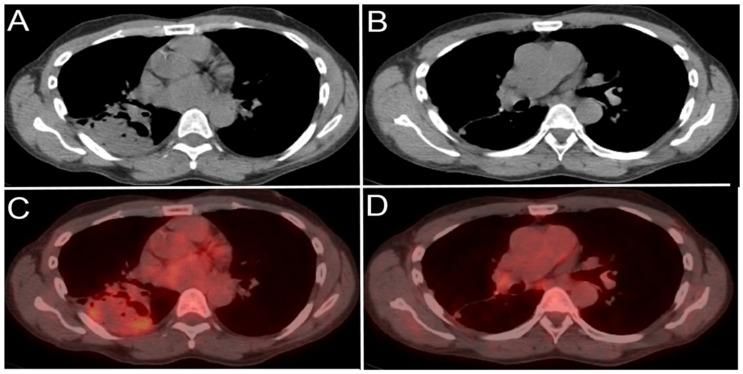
A 65-year-old male patient with right lower lobe lung cancer. The pulmonary mass seen in (A) shows avid FDG uptake (SUV = 4.6) in (C). Right hilar and subcarinal lymph nodes show mild FDG uptake (SUV = 2.6), which was found later to be inflammatory due to chemotherapy, resolving on (B,D). This is an example of false positive uptake in nonmalignant tissue.
